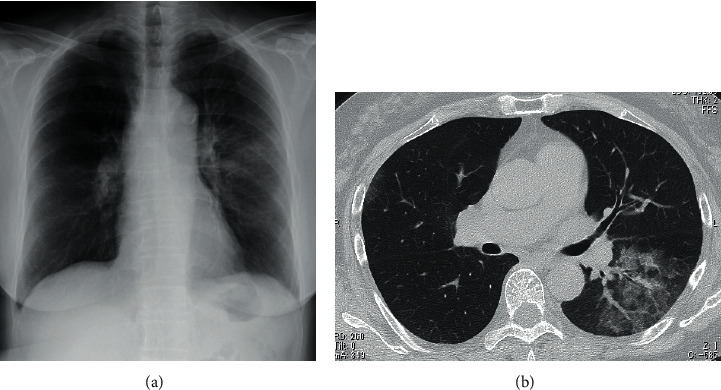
(a) Chest radiography shows subtle pulmonary infiltrate in the left lung. (b) Computed tomography (CT) reveals pulmonary infiltrates with ground-glass opacities (GGO) in the superior segment (S6) of the left lung.
